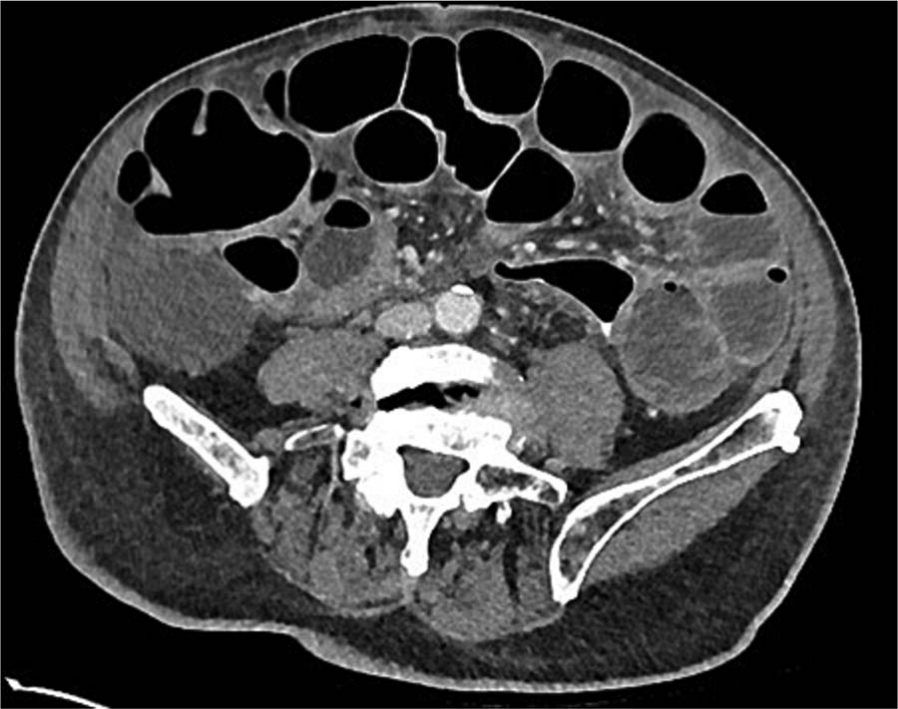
Day 7 CT scan demonstrating features consistent with ileus with no pneumoperitoneum and rectal contrast proximal to anastomosis without contrast extravasation.
